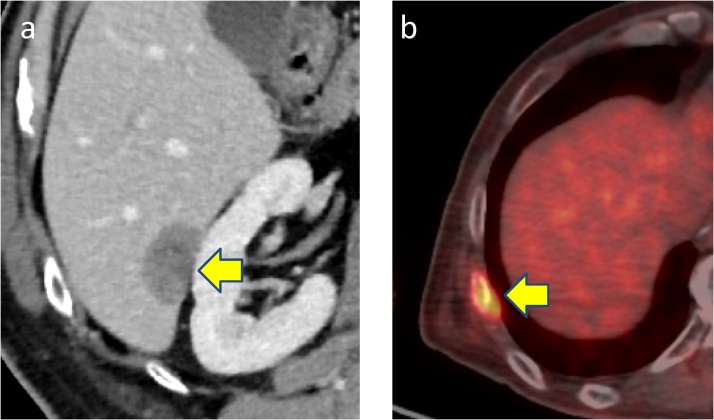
a) CT scan showed metastasis in S6 of the liver. b) PET-CT showed metastasis in the right eighth rib.
